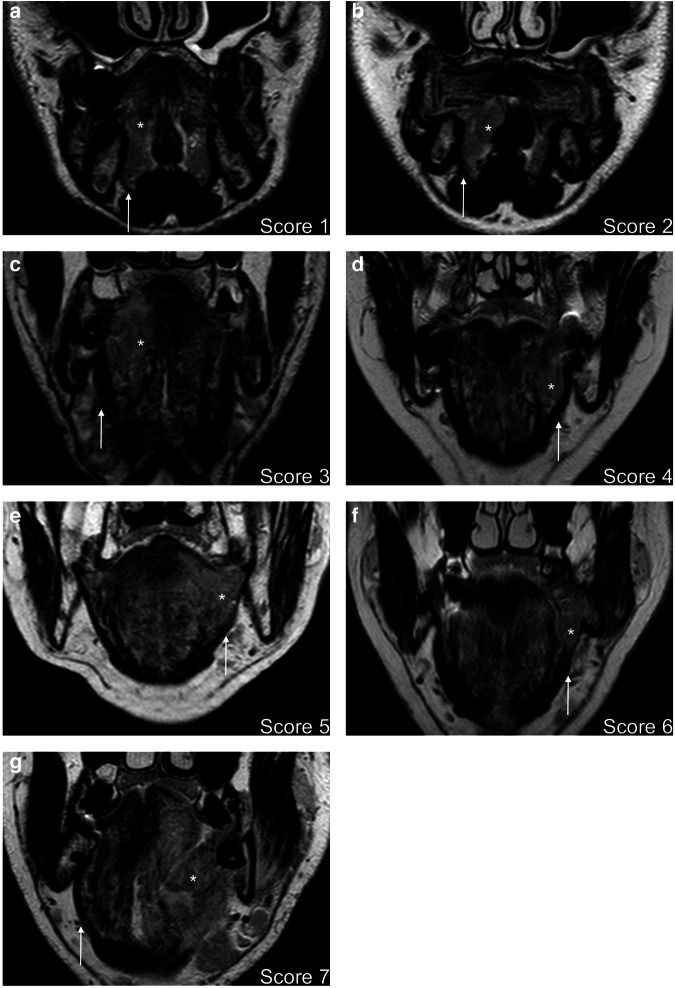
a–g We present selected T2-weighted coronal MR images from seven patients diagnosed with OSCC infiltrating the FOM. Each figure represents a specific score category in order. The tumor is identified by an asterisk in the images, while the MM’s profile is marked with an arrow (please note that in g, as the normal anatomy of the left FOM is subverted, the arrow points to the contralateral side of MM)
